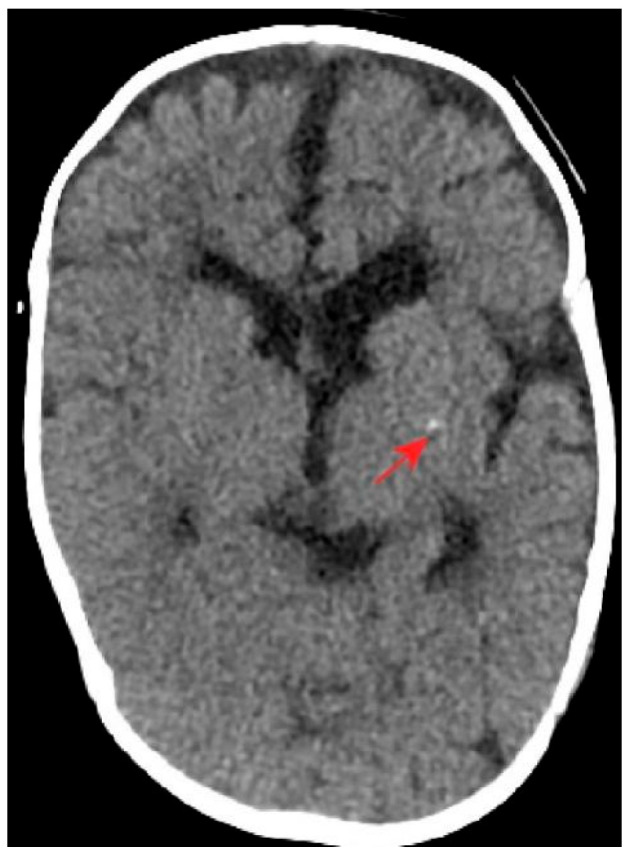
CT imaging of the brain. The arrow indicates basal ganglia calcifications.
